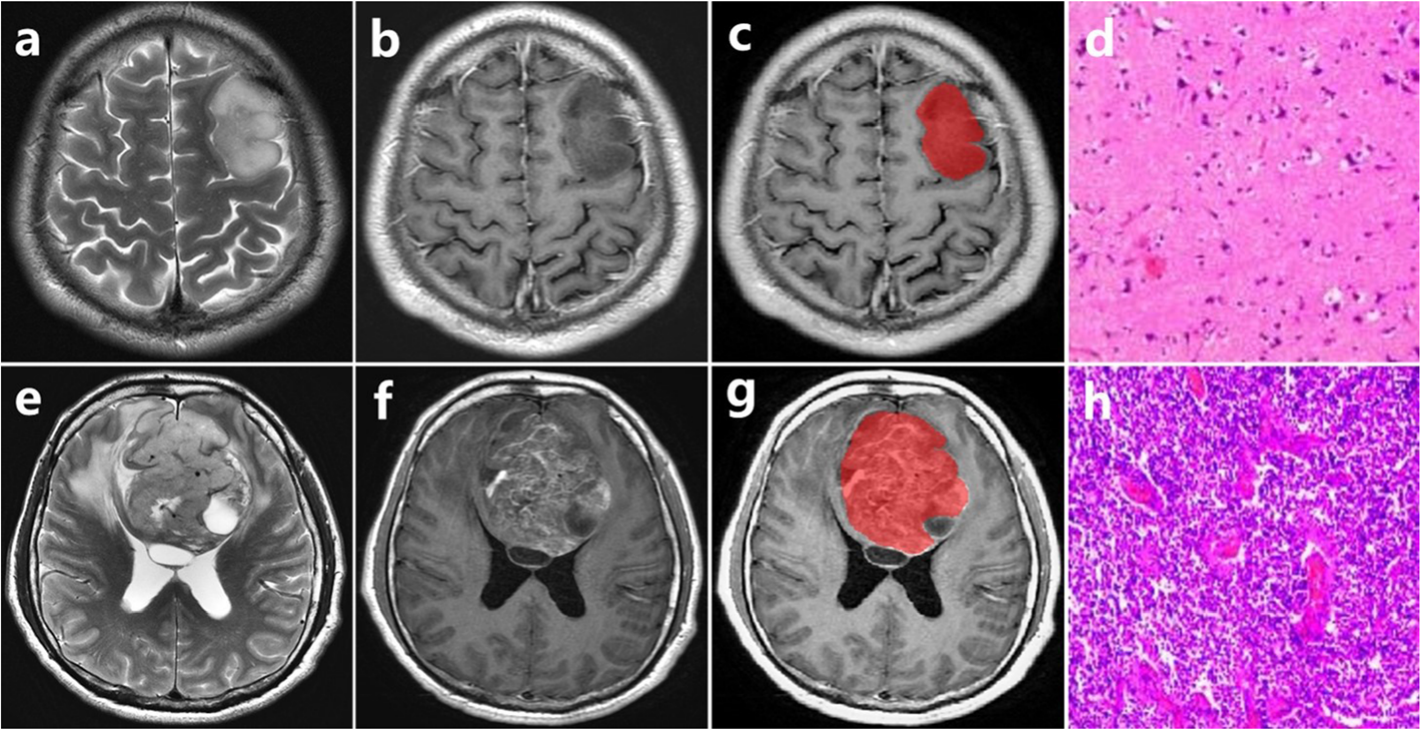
Upper row: ODG2 in the left frontal lobe from 33-year-old man; lower row: ODG3 in the bilateral frontal lobe from 46-year-old man. a, e T2-weighted image. b, f T1-weighted contrast-enhanced image. c, g The volume of interest of manually drawn. d, h Pathology slice images show cell density and vascular proliferation
